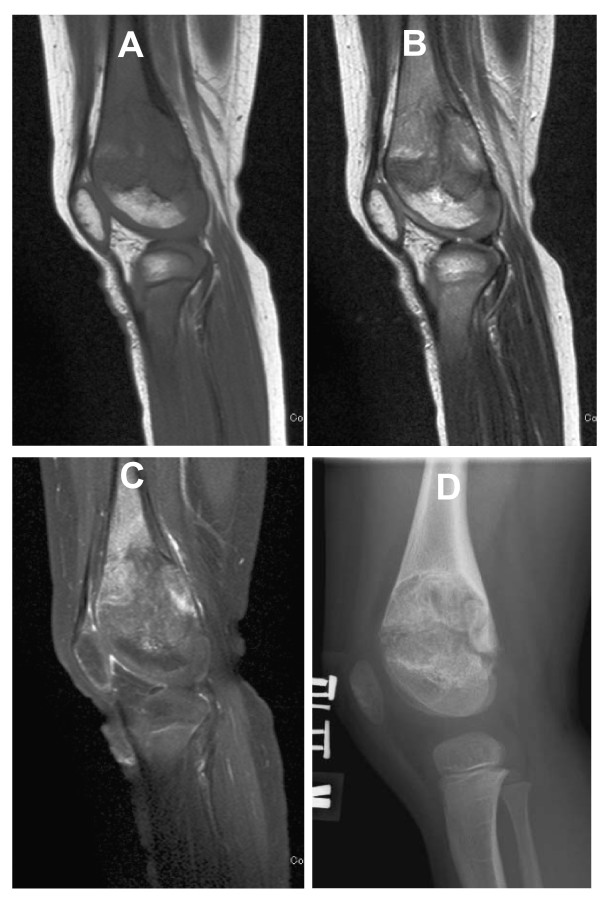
MR images and radiograph of the left knee at the age of 4 years and 3 months, after more than 20 months of anakinra treatment. (MRI A: T1-weighted, B: MRI T2-weighted, C: MRI T1-weighted with fat suppression and Ga enhancement, D radiograph). The growth plate widening previously seen (Figure 2) has alleviated with anakinra treatment. A gadolinium-enhanced MR image demonstrates less conspicuous enhancement at the chondroosseous junction after the anakinra treatment.
